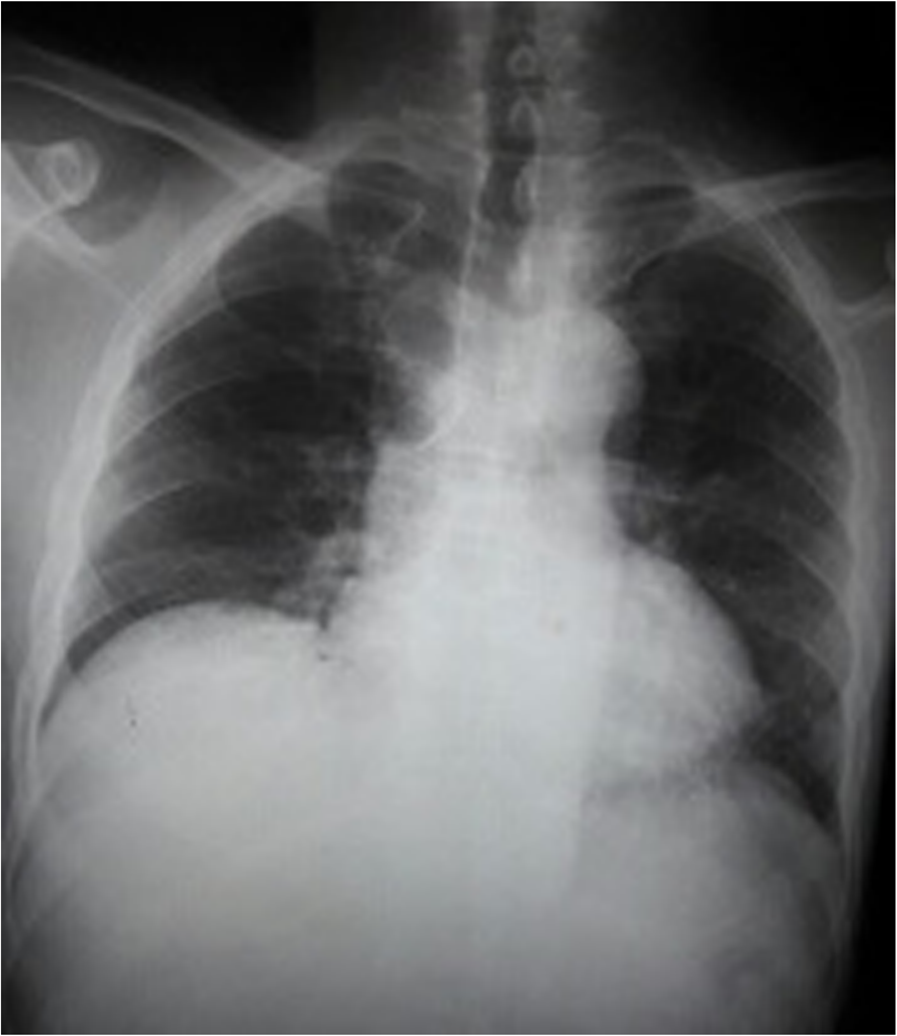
Chest X-ray of the patient, posteroanterior view
